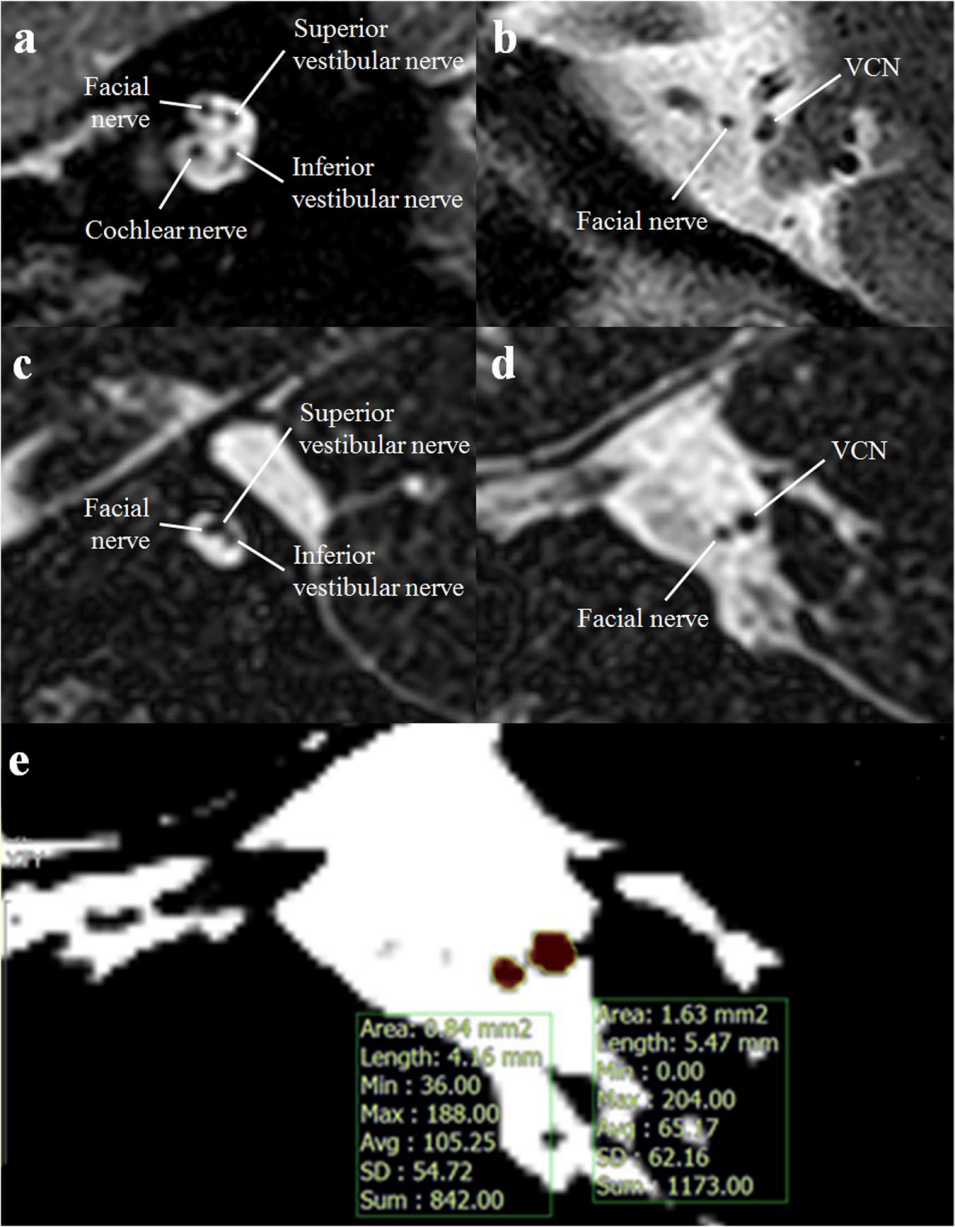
Highly contrasted T2-weighted magnetic resonance images of the internal auditory canal and cerebellopontine angle in patients with cochlear nerve deficiency (CND). (a) The image shows four normal sized nerves in the internal auditory canal, indicating cochlear nerve, facial nerve, superior and inferior vestibular nerve at each. (b) At the level of cerebellopontine angle, facial nerve and vestibulocochlear nerve (VCN), that emerge from the pontomedullary junction of mid brain, are found. The size of VCN is normal and shows a dumbbell shape. (c) The image shows only three filling defect signals in the internal auditory canal, indicating facial nerve, superior and inferior vestibular nerve at each. The cochlear nerve is not found in internal auditory canal, and the patient is diagnosed with CND. (d) A small sized VCN with a round shape is identified at the level of cerebellopontine angle in the patients with CND. (e) With an image analysis tool, the area of facial nerve and VCN are evaluated (yellow line), and the size of cochlear nerve is indirectly evaluated with the area ratio of VCN to the facial nerve.
